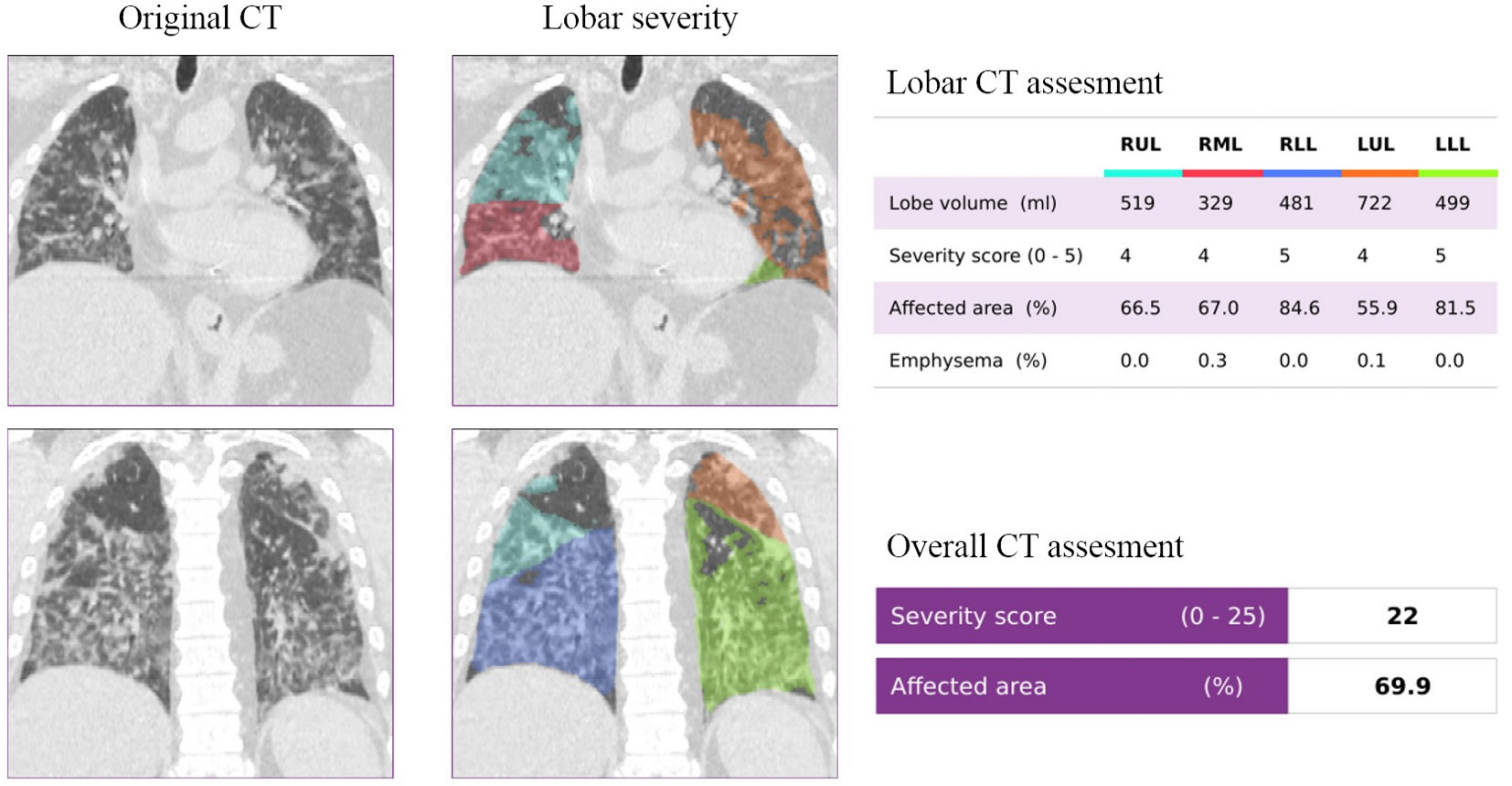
Representative example of a COVID-19 patient with a severity score of 22. The original and DL-assessed chest CT of a 76-year-old male patient, who had a positive RT-PCR test at the time of the CT examination.
